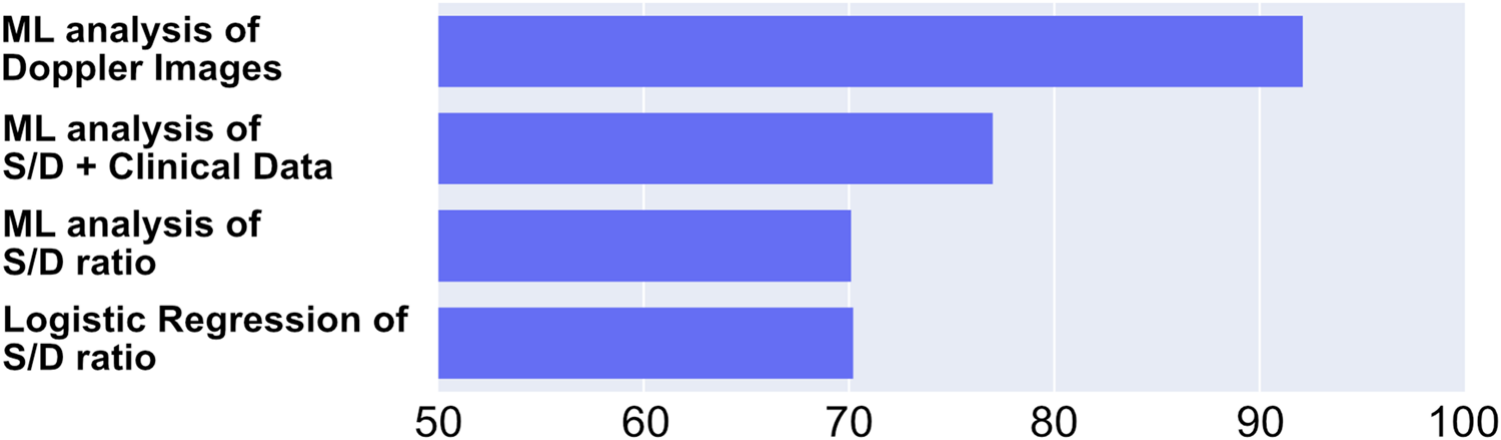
The sonographic images distinguish between IUGR fetuses vs. control using the Xception DNN architecture (ML analysis of Doppler Images), while the systolic/diastolic (S/D) ratio alone, or the S/D data and the clinical data, both have predictive performance that is markedly reduced compared to the image analysis. These data indicate that the Doppler alone contains, and ML can effectively extract, predictive information not previously available in routine clinical work
